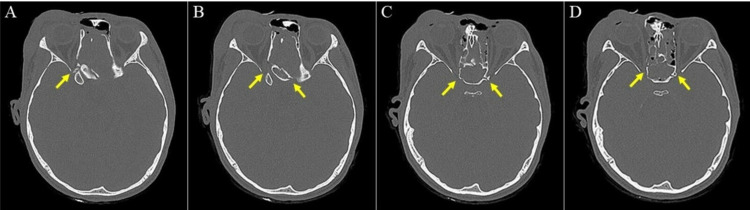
Serial plain CT images of brain and orbit. Arrows showing the oblique fracture line involving the superomedial wall of the right orbit, roof and bilateral walls of sphenoid sinus. CT: computed tomography.
